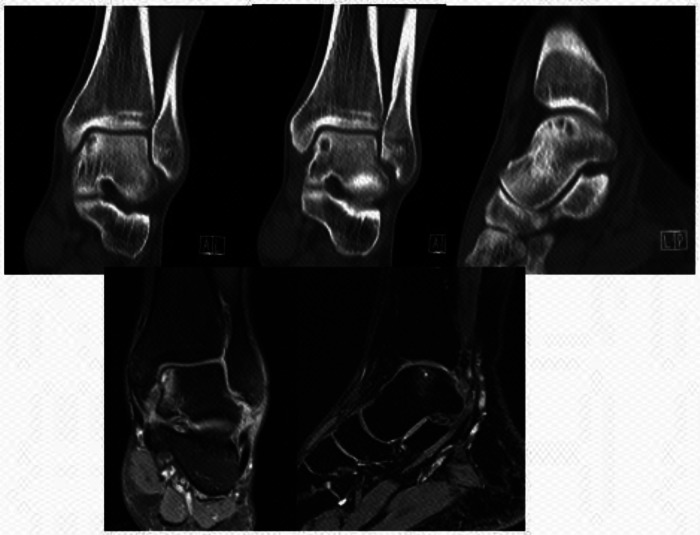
Preoperative CT and MRI image data.
